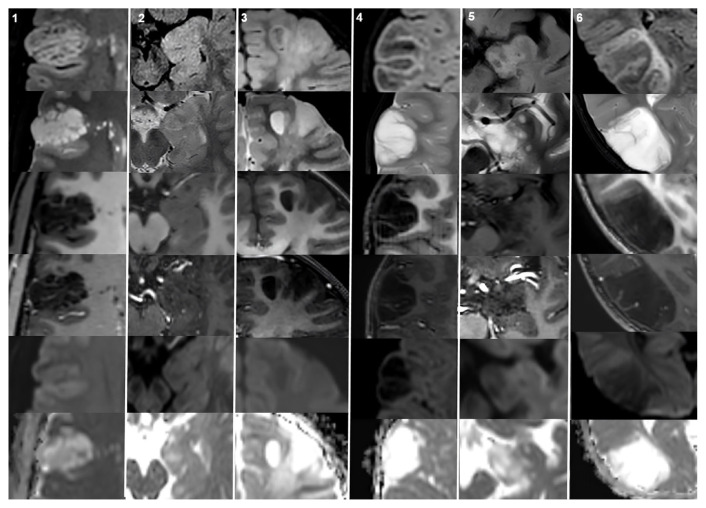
Representative DNET cases. Each column contains MRI images of different cases. All the lesions except case 2 have mixed signal intensity on FLAIR represented as high signal intensity with suppression of some internal areas. The lesion in case 2 has a hyperintense signal on FLAIR. Additionally, the FLAIR hyperintense rim sign was observed in cases 1, 4, and 6 (first row). All the lesions are hypointense on T1WI and hyperintense on T2WI (third and second row, respectively). None of the lesions in these six cases showed contrast enhancement on postcontrast T1WI (fourth row). On DWI, the lesions in cases 4 and 6 are hypointense, while the lesions in the remaining cases are isointense compared to the adjacent brain parenchyma (fifth row). No hypointensity was observed on the ADC maps in any of the lesions (sixth row).
